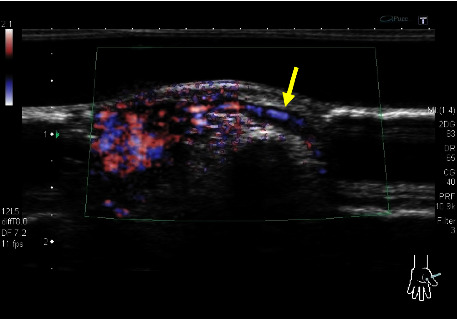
Ultrasound image of vessels flowing in and out of a tumour (yellow arrow).
